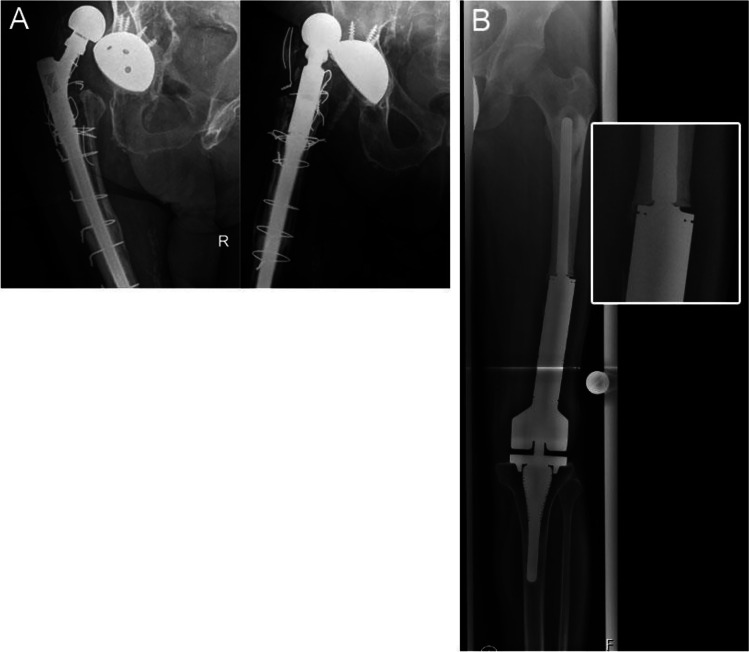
X-rays of dislocated revision THA and implant breakage of distal femur replacement. A A 70-year-old patient with dislocation of revision THA. Last dislocation occurred 5 years before index surgery. The implant was revised due to periprosthetic fracture 10 years ago. Primary arthroplasty was performed 11 years ago due to aseptic necrosis of the femoral head. B A 30-year-old patient with implant breakage of a distal femur replacement 10 years after treatment for osteosarcoma. The breakage in the junction to the socket is enlarged
